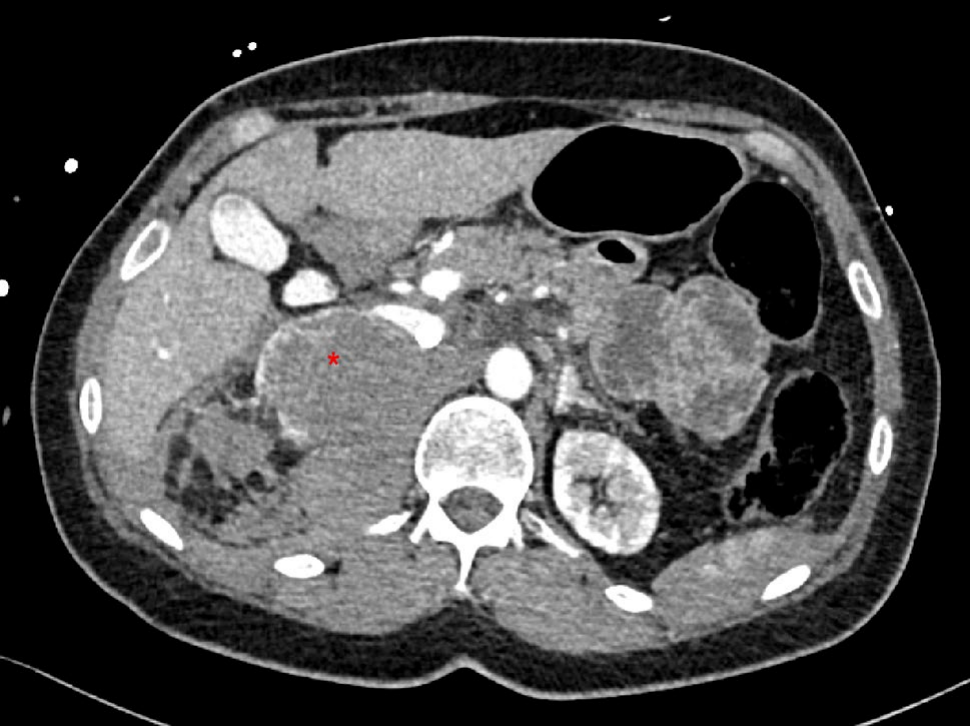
CT scan after providing sufficient circulation with VA-ECMO. *Is marking the 5 × 5 cm mass of the right adrenal gland
